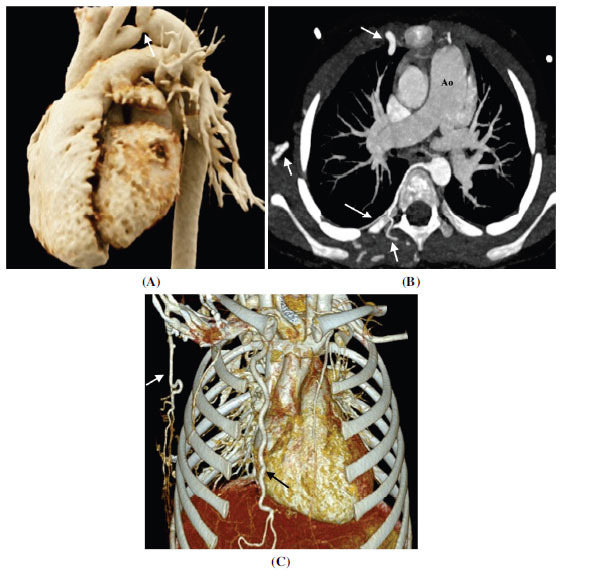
Preductal aortic coarctation. (A) Volume rendered technique image showing focal narrowing of the aortic lumen at the left subclavian artery origin and just proximal to it. (B) Axial contrast-enhanced computed tomographic angiography image and (C) Volume rendered technique image showing enlarged rights subscapular, right internal mammary, and posterior intercostal arteries providing collateral circulation to descending thoracic aorta. Note that the collateral circulation is provided through the ride side only as the aortic narrowing is proximal to the left subclavian artery.
